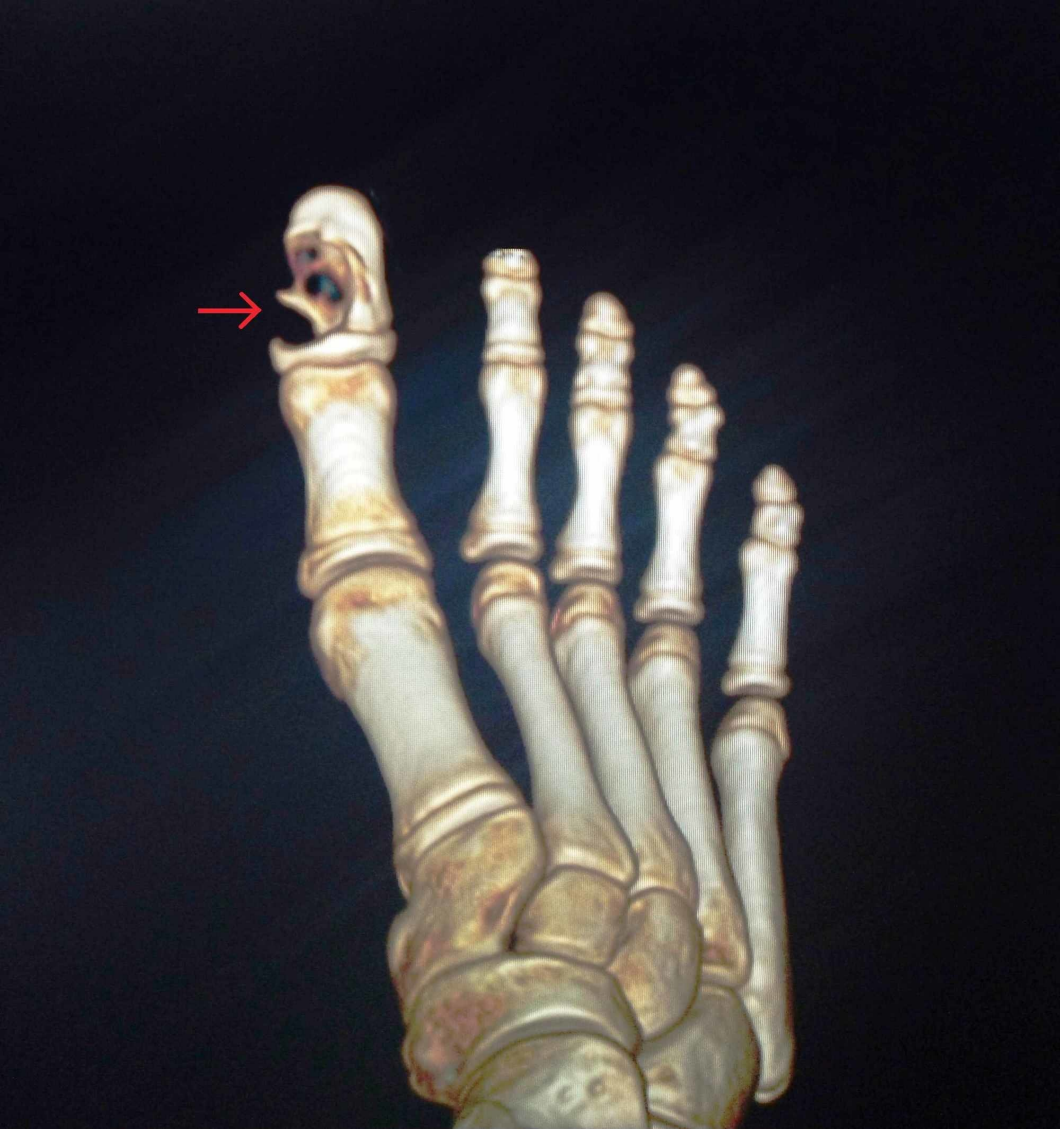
3D-reconstructed computed tomography image of the right foot The image shows lytic lesions with internal septations
